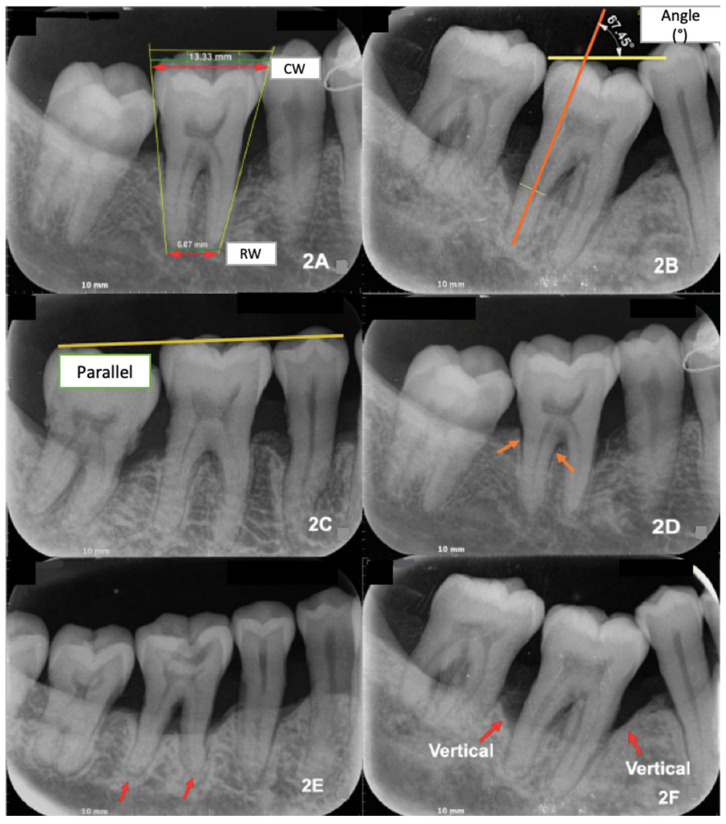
Radiographic parameters evaluated in mandibular first molars. (A) Crown width (CW) and root width (RW) with root convergence (occlusal vs. apical third). (B) Tooth axis inclination relative to occlusal plane (°). (C) Occlusal arch alignment (parallel vs. non-parallel) (D) Periodontal ligament (PDL) space widening and lamina dura thinning. (E) Root morphology (normal, blunted, or curved roots). (F) Pattern of alveolar bone loss (horizontal vs. vertical).
